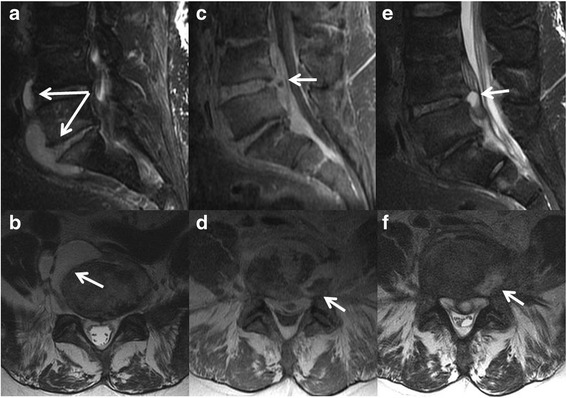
Initial lumbar spine magnetic resonance imaging performed without gadolinium demonstrated extensive abnormal T2 hyperintensity of the L5 interspace (a and b, sagittal fat-saturated T2-weighted image and axial T2-weighted image at the L5 interspace) indicating discitis. A large multilobulated fluid collection was contiguous with the infected interspace indicating an abscess (*arrow*, a and b). Follow-up lumbar spine magnetic resonance imaging performed with gadolinium demonstrated extensive abnormal enhancement (c and d, post-gadolinium sagittal T1-weighted with fat saturation and axial T1-weighted images, respectively) and T2 hyperintensity (e and f, sagittal T2-weighted with fat saturation and axial T2-weighted at the L4 interspace) involving the L3 to L4, L4 to L5, and L5 to S1 interspaces. There is abnormal paraspinal and epidural enhancing phlegmon with a focal ventral epidural abscess (*arrow*, c and e) emanating from the L4 to L5 interspace. An additional abscess involving the left posterolateral soft tissues (*arrow*, d and f) was targeted during biopsy and yielded 10 cc of fluid
